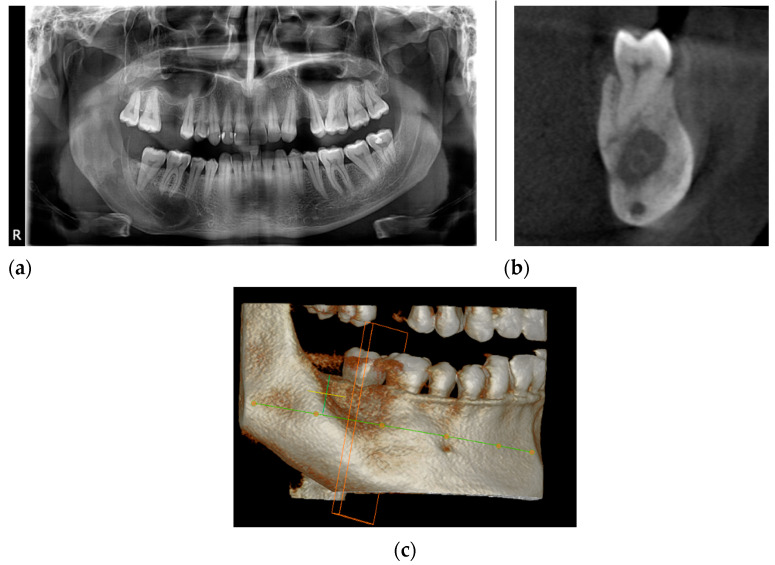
The radiological aspect after one year of decompression: (a) panoramic radiography shows a reduction in the size of the cyst; (b) the CBCT, coronal view indicates the position of the tube in the cyst cavity; (c) the CBCT, 3D reconstruction view.
